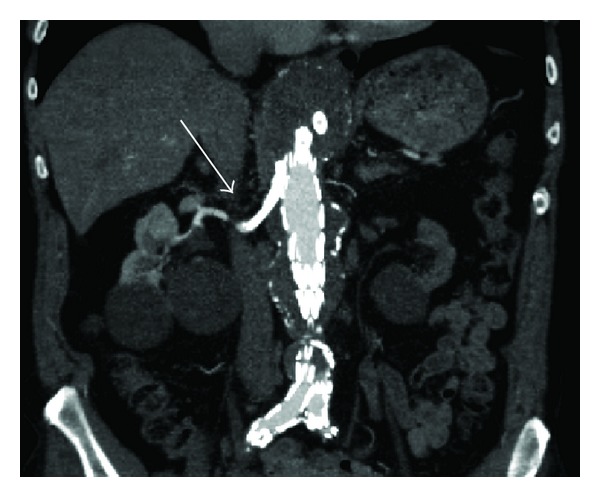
Postoperative CTA showing adequate position of the aortic branch graft with patent side branch in the right renal artery (arrow).
